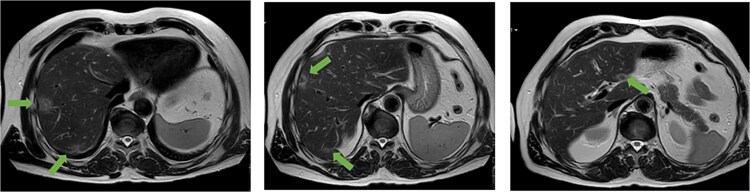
Axial MRI slices obtained preoperatively showing bilobar colorectal liver metastases. Key lesions are marked with arrows.
